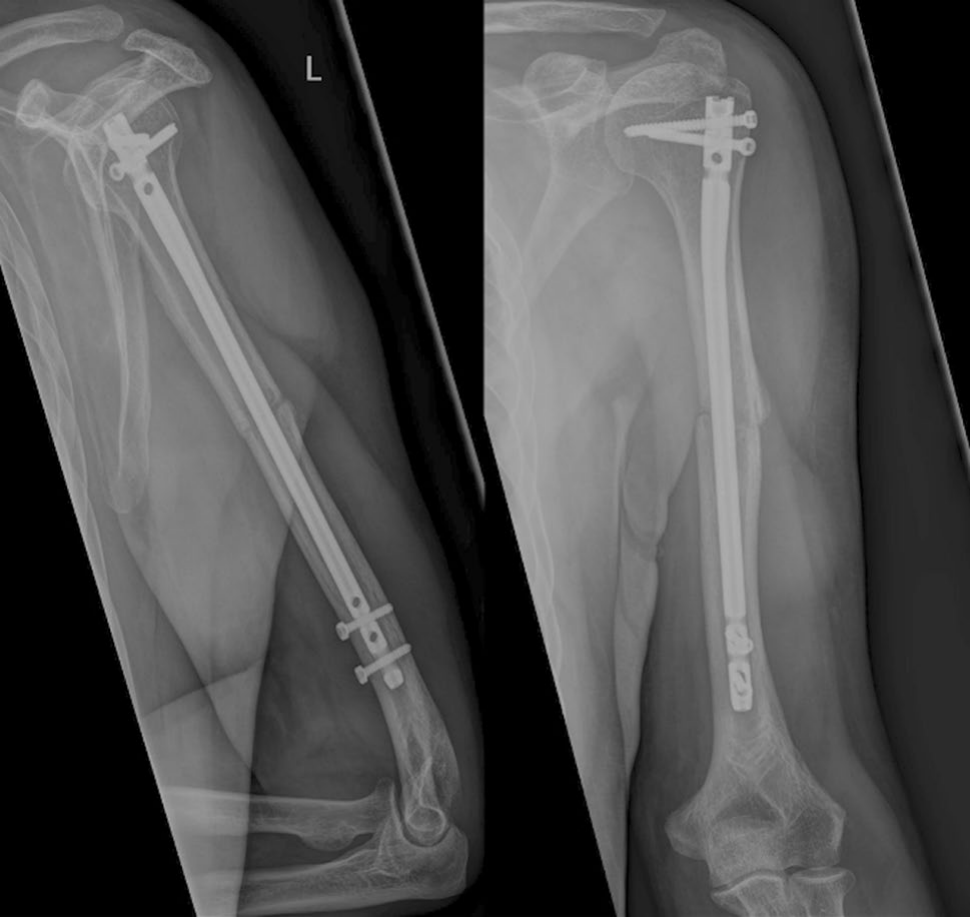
postoperative a.p. and lateral radiograph showing anterior–posterior locking technique
